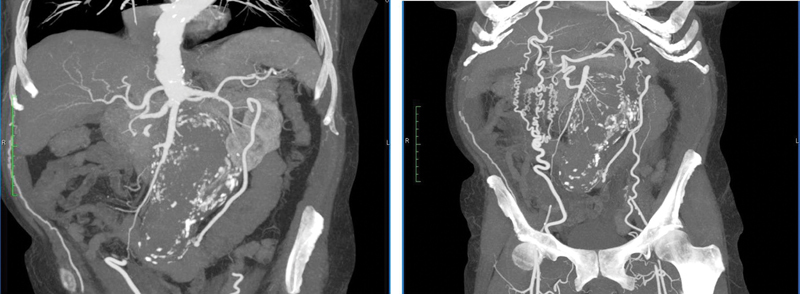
A computed tomography (CT) angiography 10 days after the first CT revealed an acute thrombosis of abdominal aortic aneurysm just below both the renal arteries with rich collateralization between the thoracic aorta and the common femoral arteries through the superficial epigastric arteries and other arteries of thoracic and abdominal wall.
